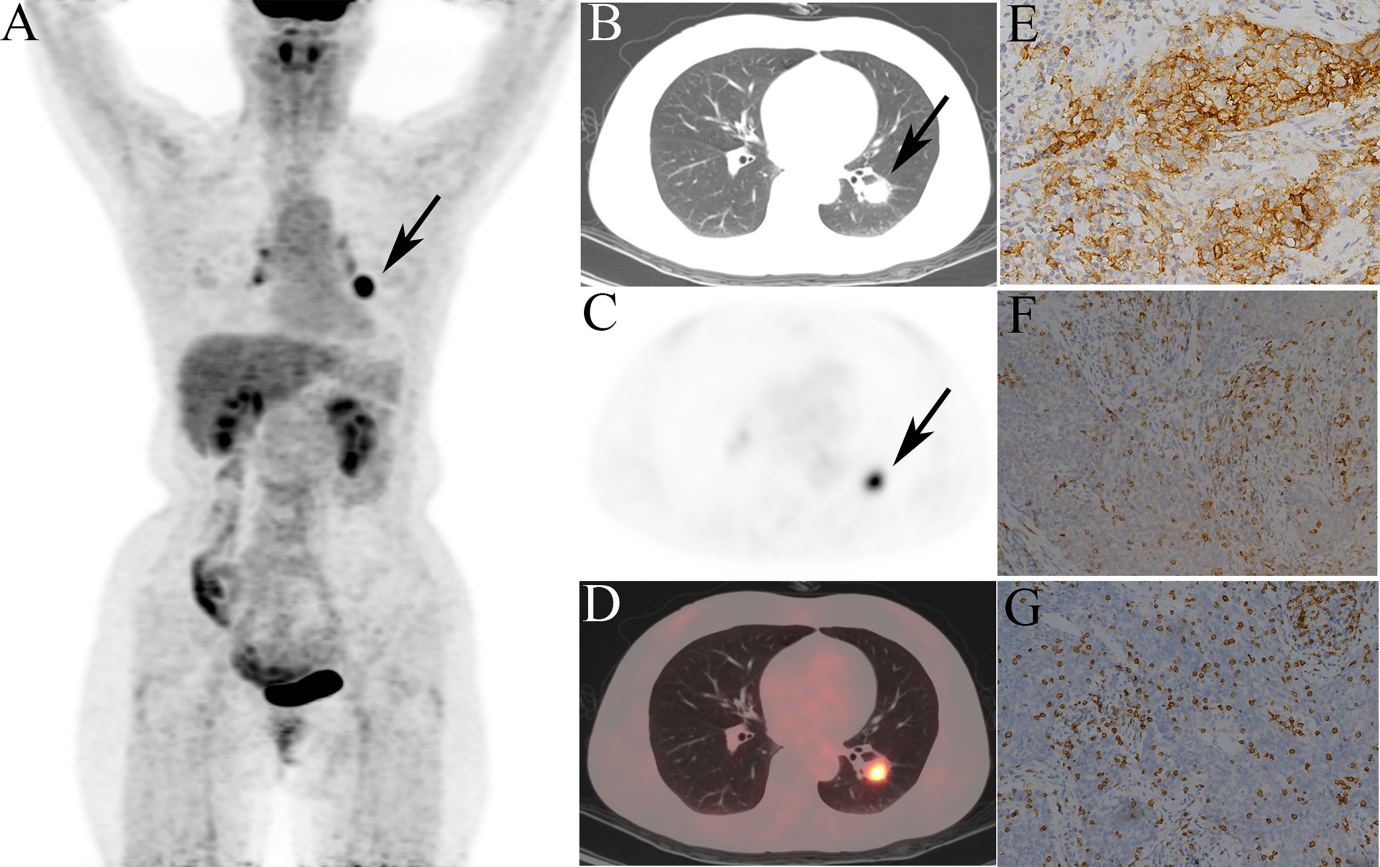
Representative ^18^F-fluorodeoxyglucose positron emission tomography/computed tomography (^18^F-FDG PET/CT) images of a 52-year-old female patient, defined as tumor microenvironment immune type (TMIT)-I tumor. (A) MIP figure, (B–D) CT, PET, PET/CT fusion images. A nodule was in the lower lobe of the left lung (arrow) with markedly increased radioactivity, SUVmax: 10.3, GLRLM_LRHGE: 382, GLZLM_SZE: 0.682, and GLCM_Contrast: 14.6. The surgical pathology: poorly moderately differentiated squamous cell carcinoma. (E) High programmed death-ligand 1 (PD-L1) expression. (F) Programmed death-1 (PD-1) tumor-infiltrating lymphocytes (TILs) high density. (G) CD8+ TILs high density.
